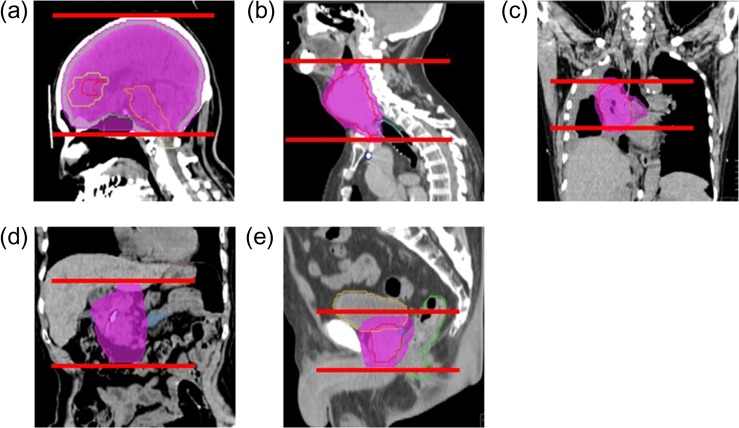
CT images and scan areas of five clinical cases for evaluating the organ dose from MVCT delivery: (a) brain, (b) head and neck, (c) lung, (d) abdomen (pancreas) and (e) prostate. The scan areas are from the superior edge to the inferior edge of the planning target volume (PTV, magenta area).
